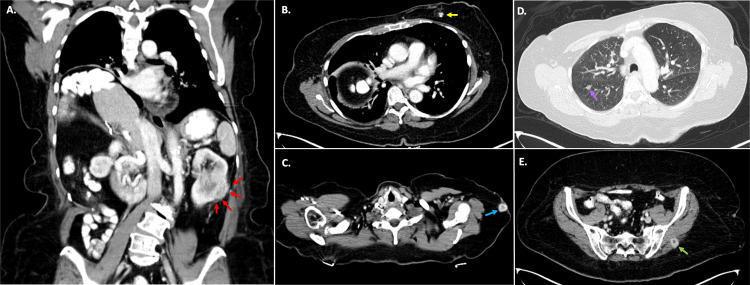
Contrast-Enhanced CT of the Chest, Abdomen, and Pelvis (A) Renal cell carcinoma primary lesion (red arrows), a large, heterogeneously enhancing mass invading the lower pole of the left kidney but which did not appear to involve the inferior vena cava, the left renal vein, or the left renal collecting system. (B, C) Left breast metastatic lesion (yellow arrow), which was first observed on screening mammogram, and the left axillary subcutaneous metastasis (blue arrow), which was discovered on ultrasound exam when the patient presented for biopsy of her breast mass. (D, E) Additional circumscribed, enhancing metastatic lesions in the lungs (lavender arrow) and in the gluteus muscle (green arrow).
